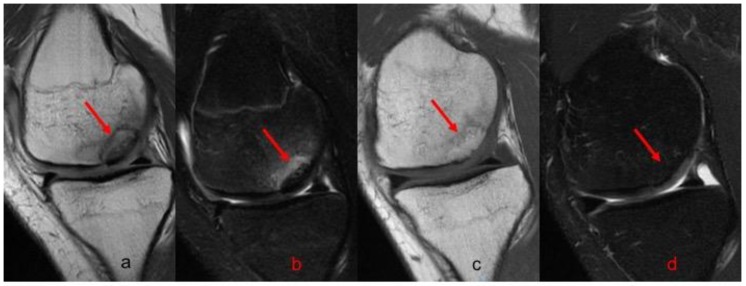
MRI evolution of grade III OCD of 2.9 cm^2^ in the medial condyle of left knee fixed with two Herbert screws. (a) T1 preoperative sagittal view; (b) T2 preoperative sagittal view; (c) Table 1. One-year postoperative sagittal view; (d) T2 1 year postoperative sagittal view. In all images the red arrow indicates the lesion.
